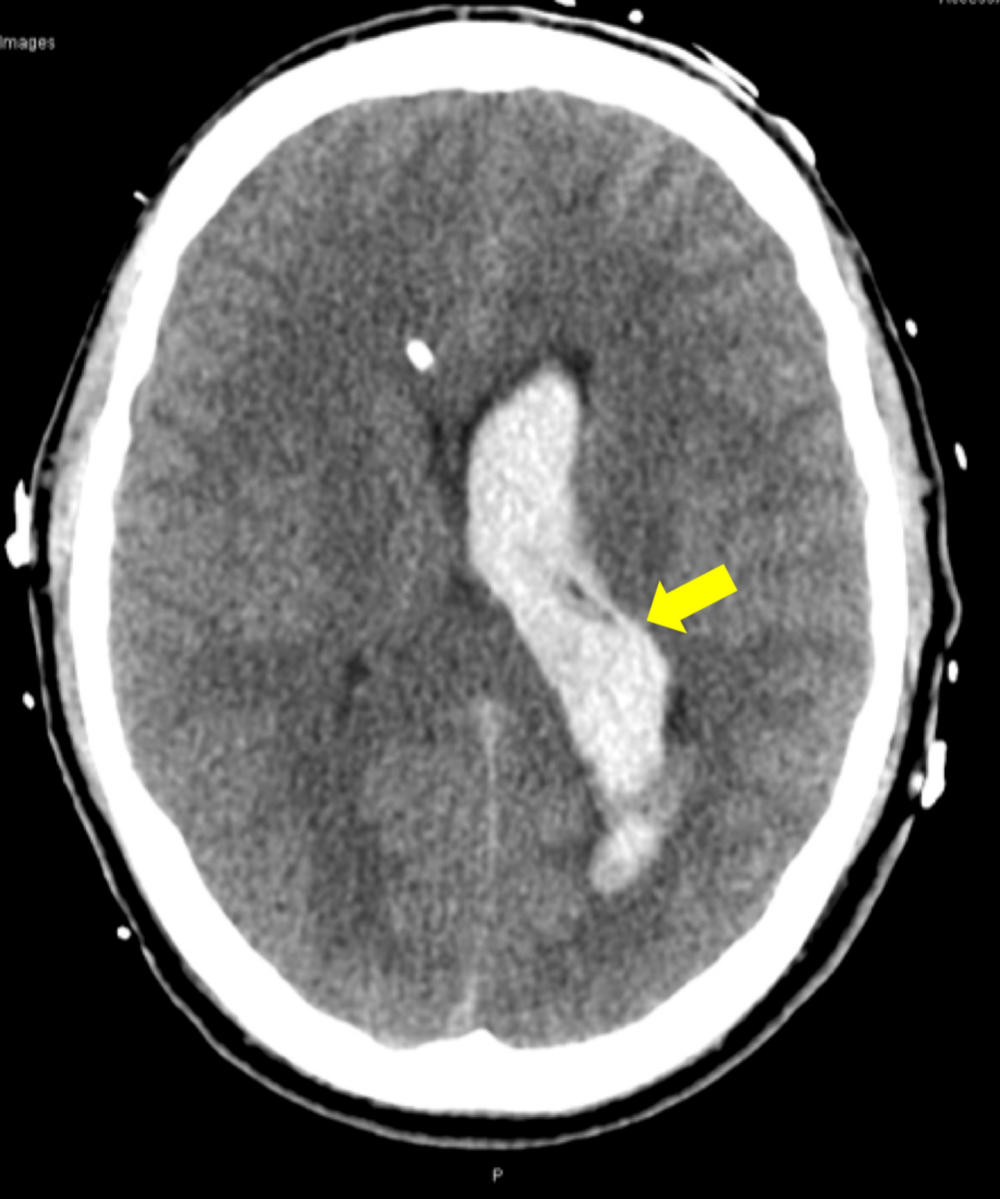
Transverse CT head image showing left intraventricular hemorrhage CT, computed tomography
